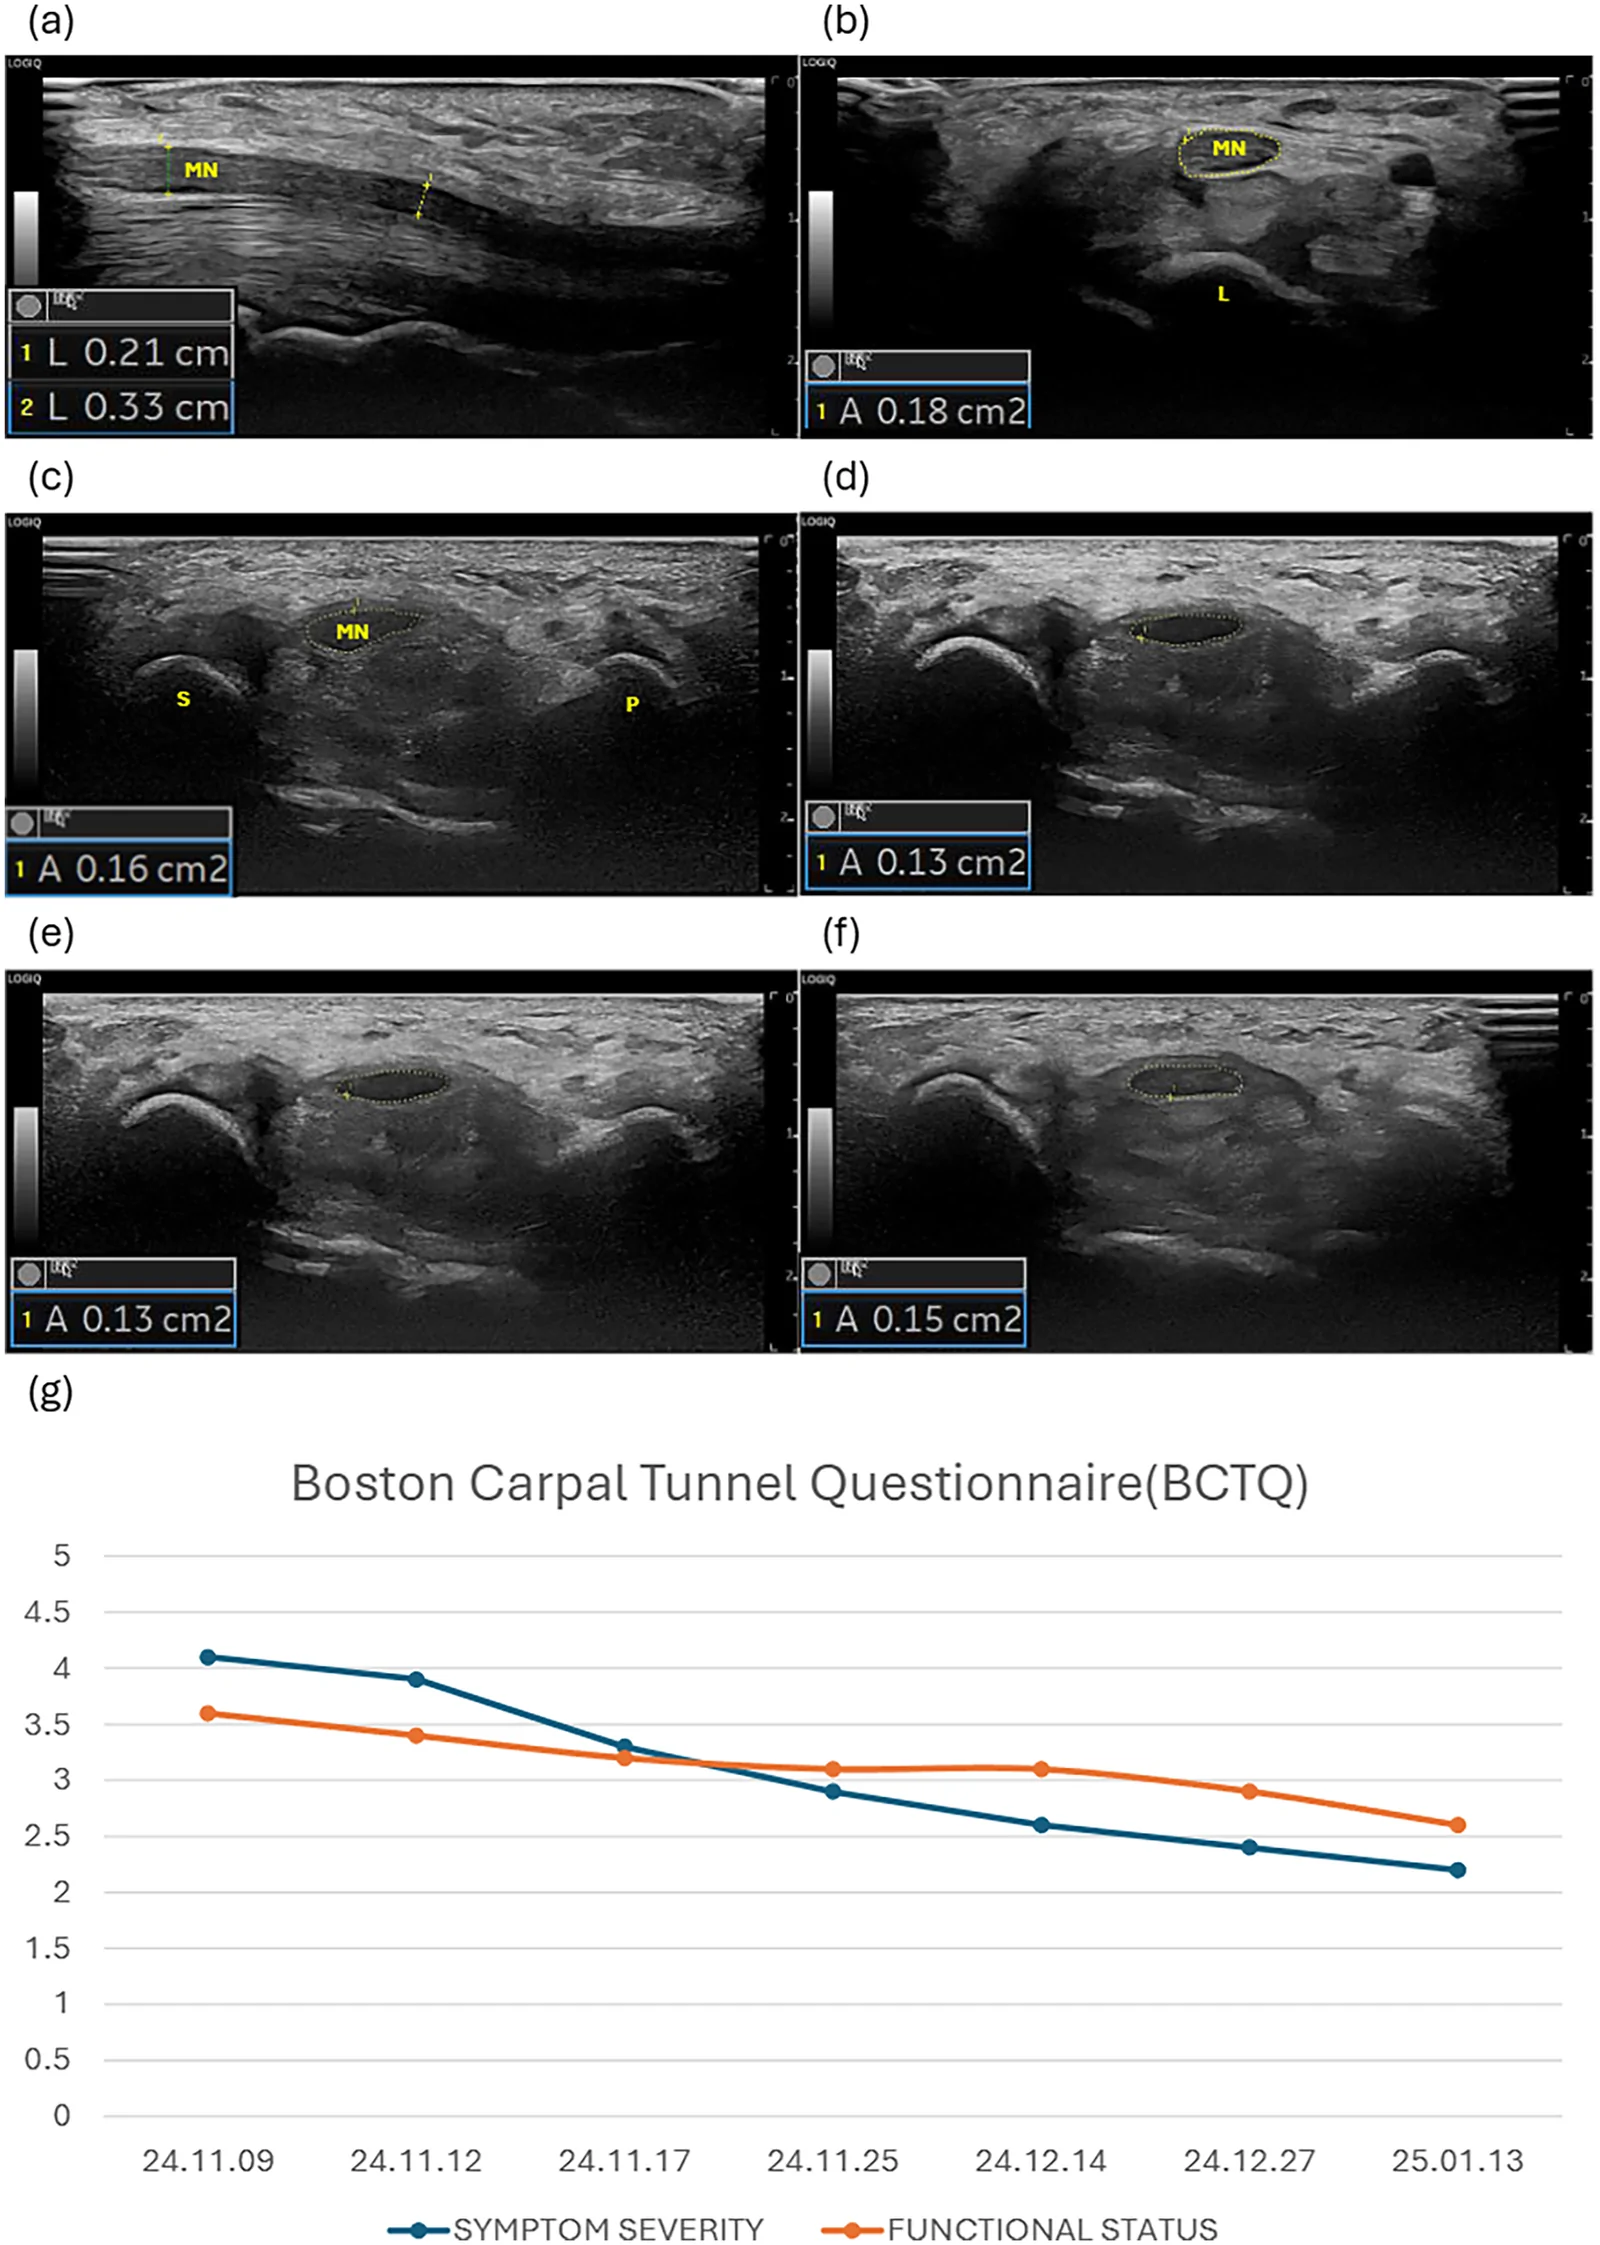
Ultrasound findings and clinical outcomes in case 1. (a) Longitudinal ultrasound image of the median nerve showing a notch sign. (b) Transverse ultrasound image at the lunate level demonstrating an increased cross-sectional area (CSA) of the median nerve (18 mm^2^). (c) Transverse ultrasound image at the proximal carpal tunnel, with the fingers in a neutral position showing the location and CSA of the median nerve. (d) Transverse ultrasound image taken during finger flexion demonstrating ventral displacement and flattening of the median nerve with a reduced CSA (16 mm^2^ → 13 mm^2^). (e) Pre-treatment ultrasound image showing the CSA of the median nerve measured at the proximal carpal tunnel (13 mm^2^). (f) Post-treatment ultrasound image demonstrating an increased CSA (15 mm^2^) and improved morphology of the median nerve after ultrasound-guided hydrodissection. (g) Changes in Boston Carpal Tunnel Questionnaire (BCTQ) scores during the treatment period, showing progressive improvement in symptom severity and functional status. CSA, cross-sectional area; L, lunate; MN, median nerve; P, pisiform; S, scaphoid.
